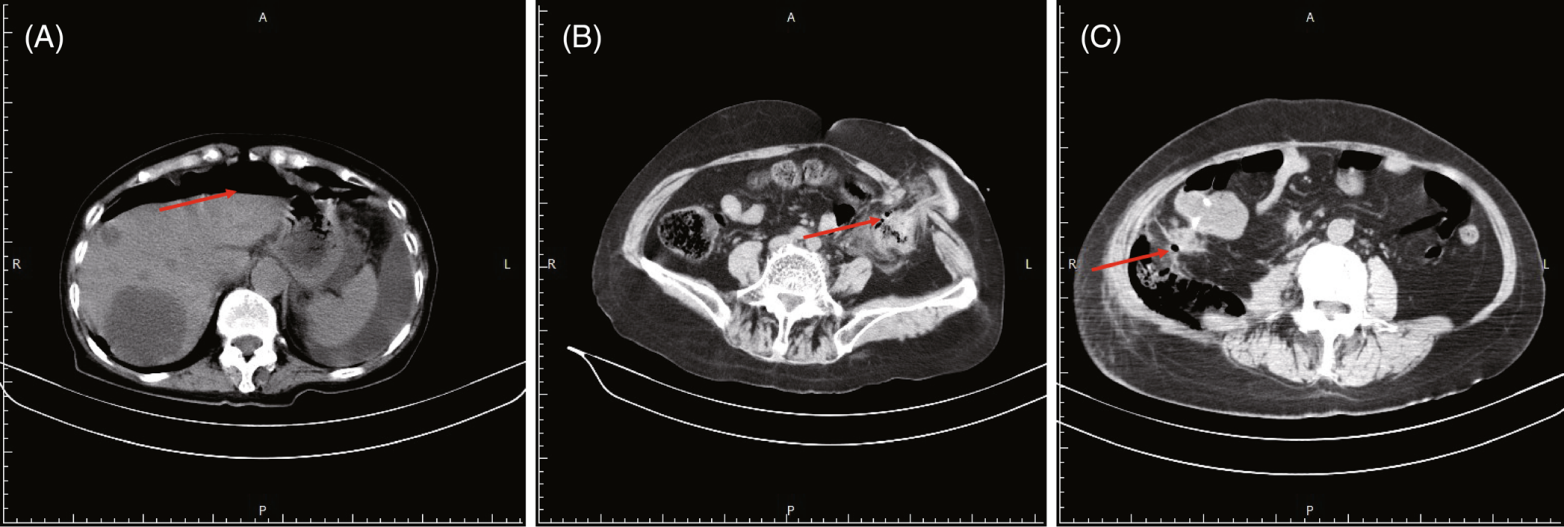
CT of intestinal perforation after receiving bevacizumab. (A) Case 1 images. CT of intestinal perforation after receiving bevacizumab. (B) Case 2 images. CT of intestinal perforation after receiving bevacizumab. (C) Case 3 images. CT of intestinal perforation after receiving bevacizumab.
